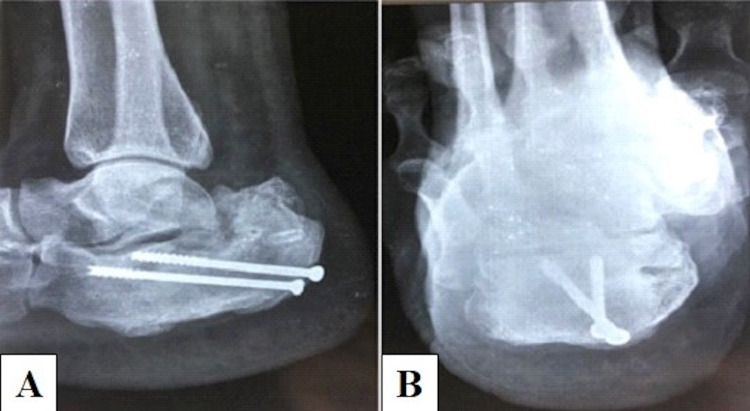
Follow-up radiographs (weight-bearing) showing union at the fracture site. (A) Lateral view. (B) Axial view.
